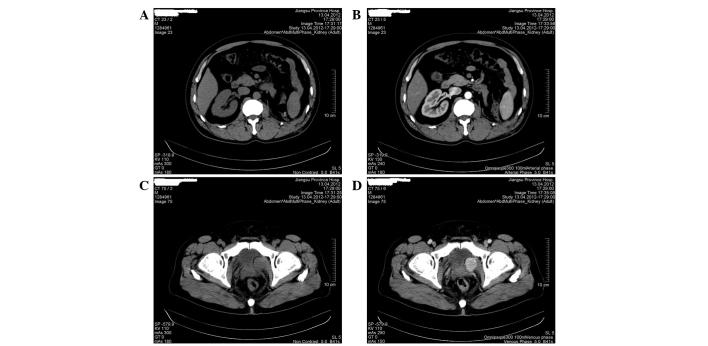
(A and B) Abdominal computed tomography scan (unenhanced) prior to the second surgery showed the absence of the left kidney without tumor recurrence or metastasis in the abdomen. (C and D) The volume of the periprostatic mass with apparent enhancement effect had evidently increased (3.5×2.8 cm^2^ at the maximum diameter) compared with that prior to the initial surgery (conrast-enhanced CT).
